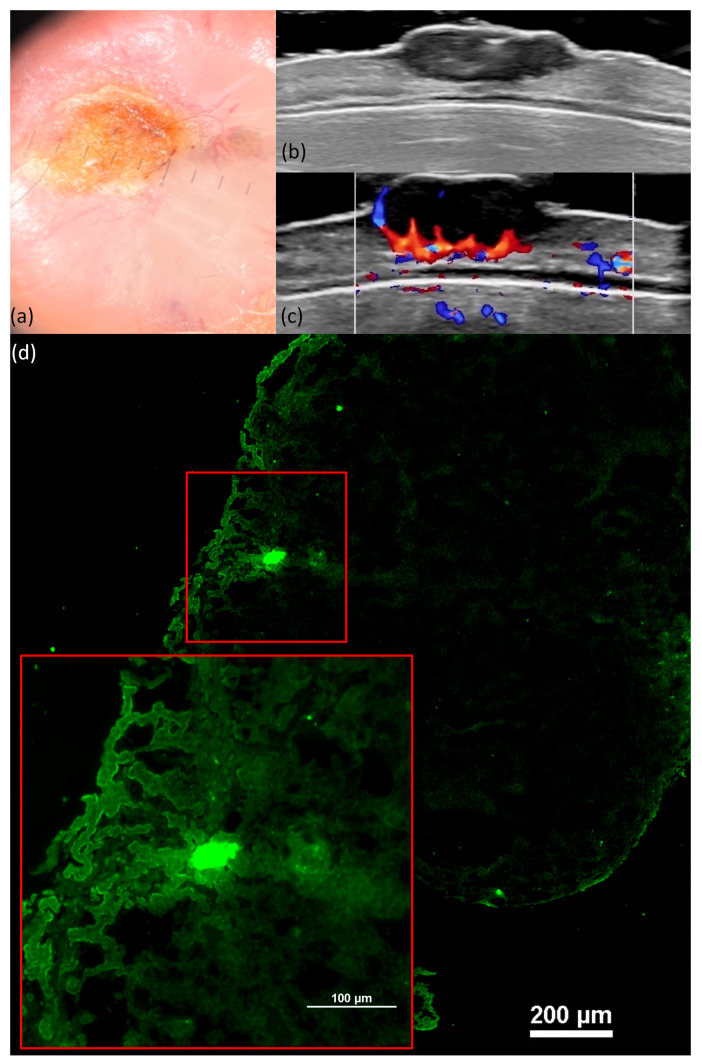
Ulcerated BCC. Dermoscopy shows arborized vessels, ulceration, multiple blue-grey globules, and shiny white-red structureless areas (a). US shows a hypoechoic lesion, imprecisely delimited, that reaches the level of the reticular dermis and contains hyperechoic points (b). Doppler mode shows an increase in tumor and peripheral vascularity (c). FCM shows well-defined tumor islands and a “cauliflower-like” appearance (d).
